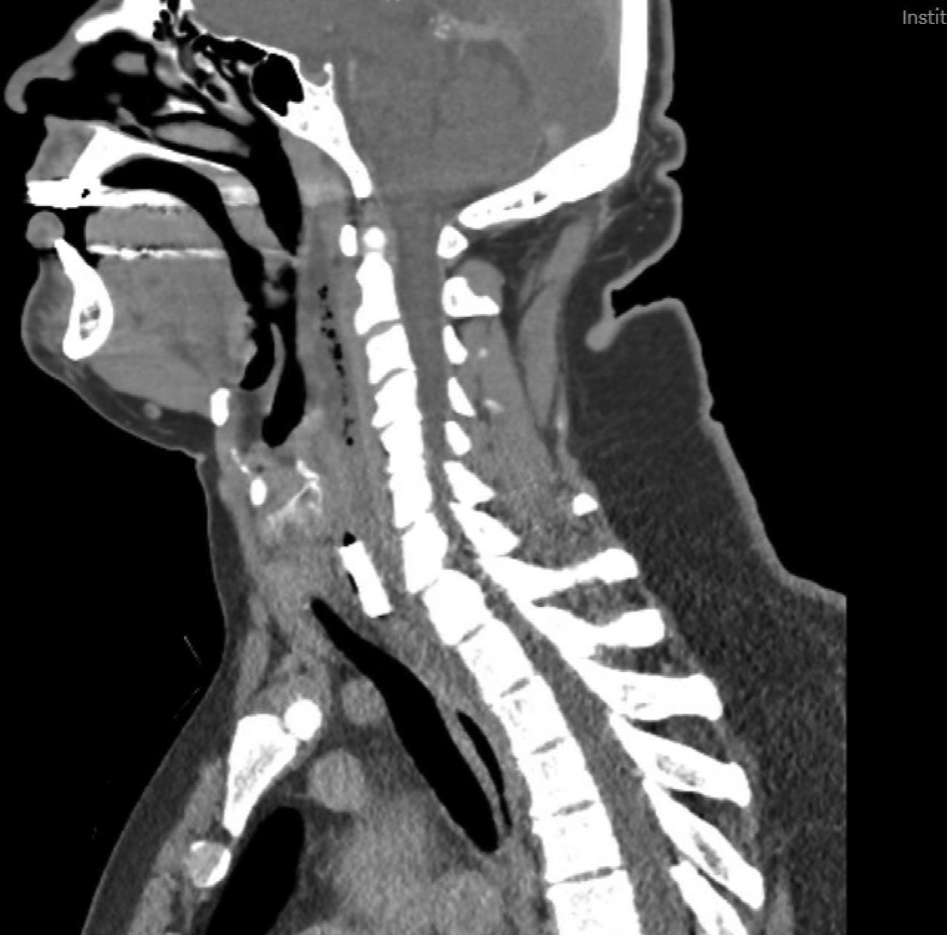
Computed tomography of the neck demonstrated a rectangular metallic density with multiple foci of gas consistent with esophageal perforation.
